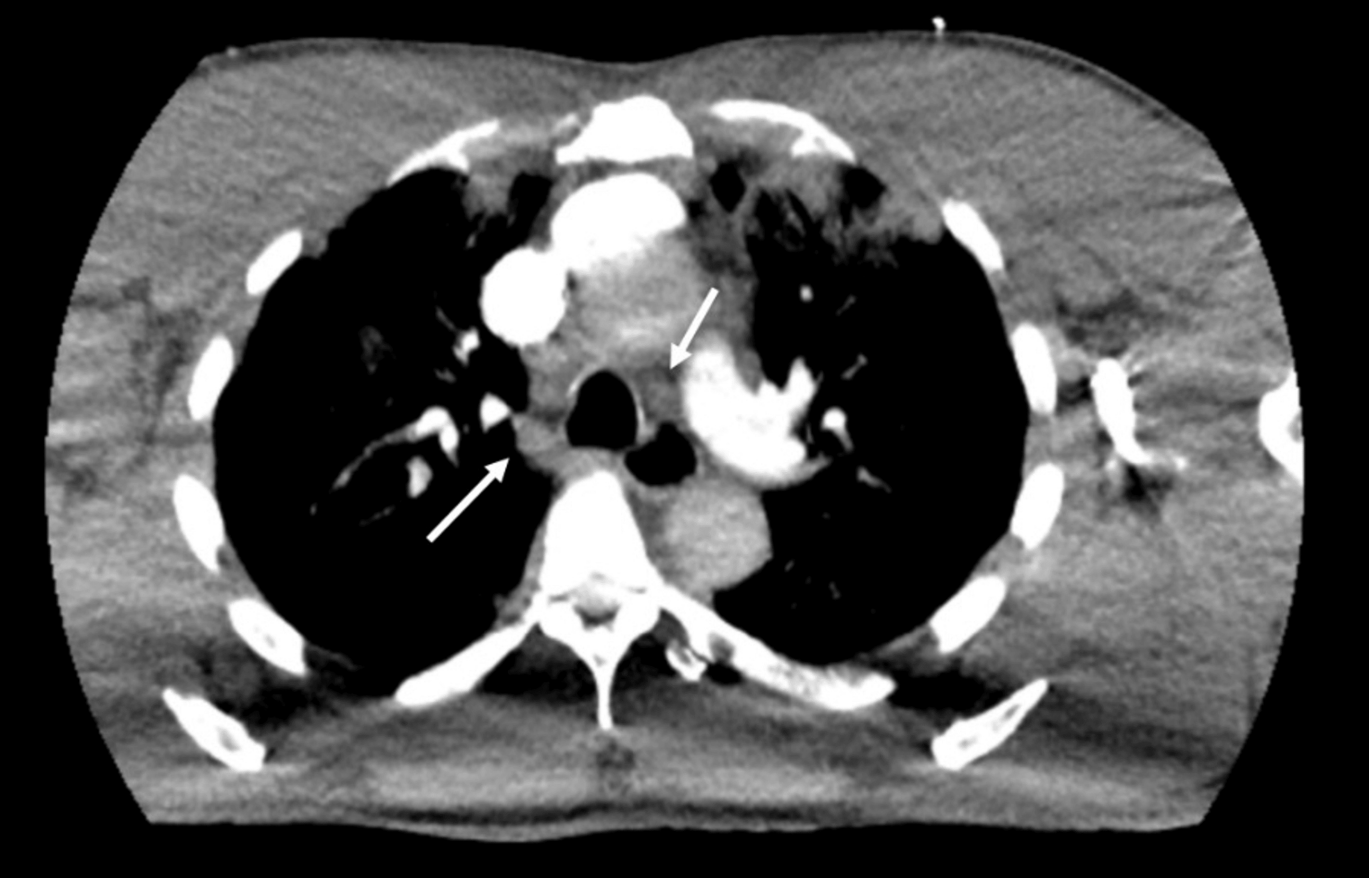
Axial CT chest images of the mediastinal window, showing bilateral lower paratracheal lymph nodes (arrows).
